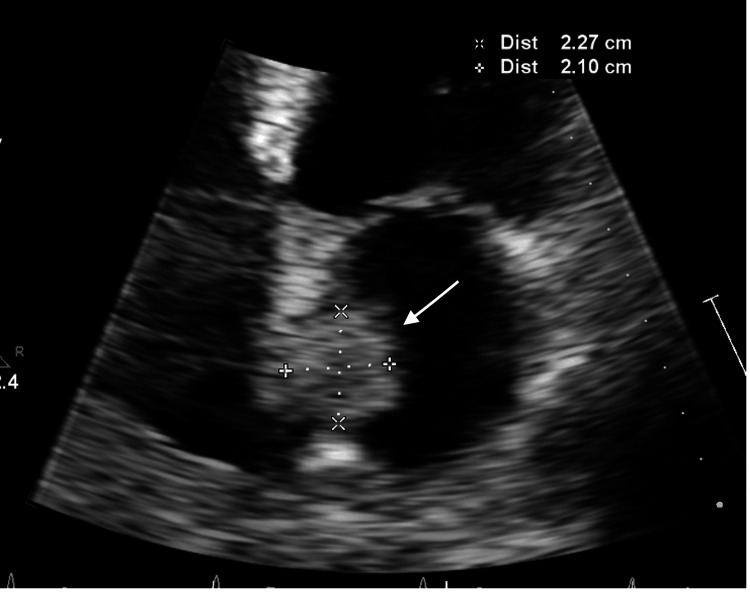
Transthoracic echocardiogram A 2.27 × 2.10 cm sessile left atrial mass (arrow) was visualized in the apical four-chamber view on transthoracic echocardiogram.
